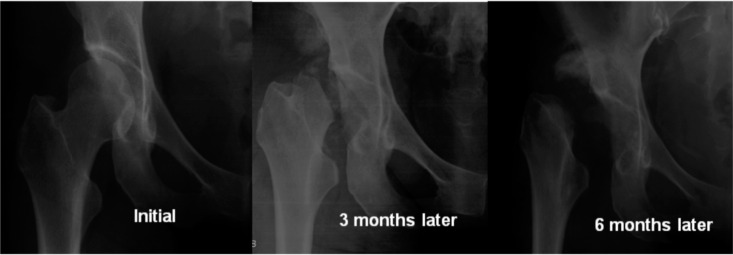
The three radiographs showed that the progression of osteolysis of the femoral head and neck within six months.
